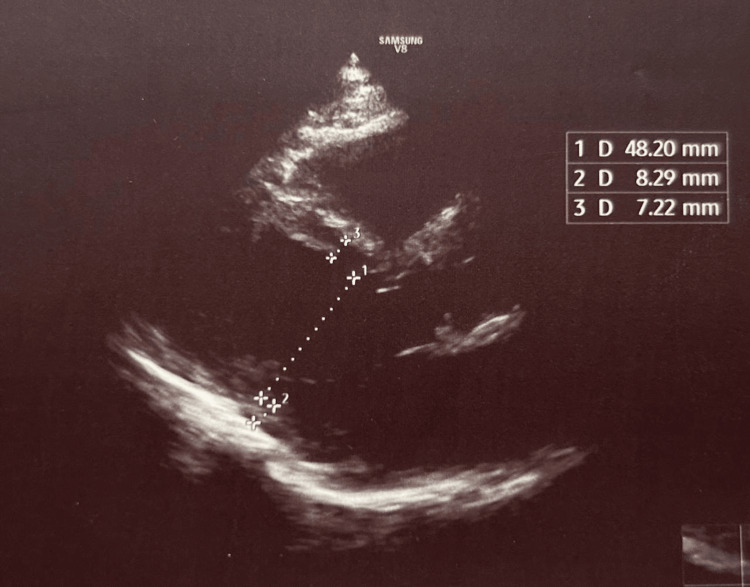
Transthoracic echocardiography showing normal cardiac morphology and function Parasternal long-axis transthoracic echocardiographic view showing normal cardiac chamber dimensions and wall thickness. Linear measurements are indicated.
